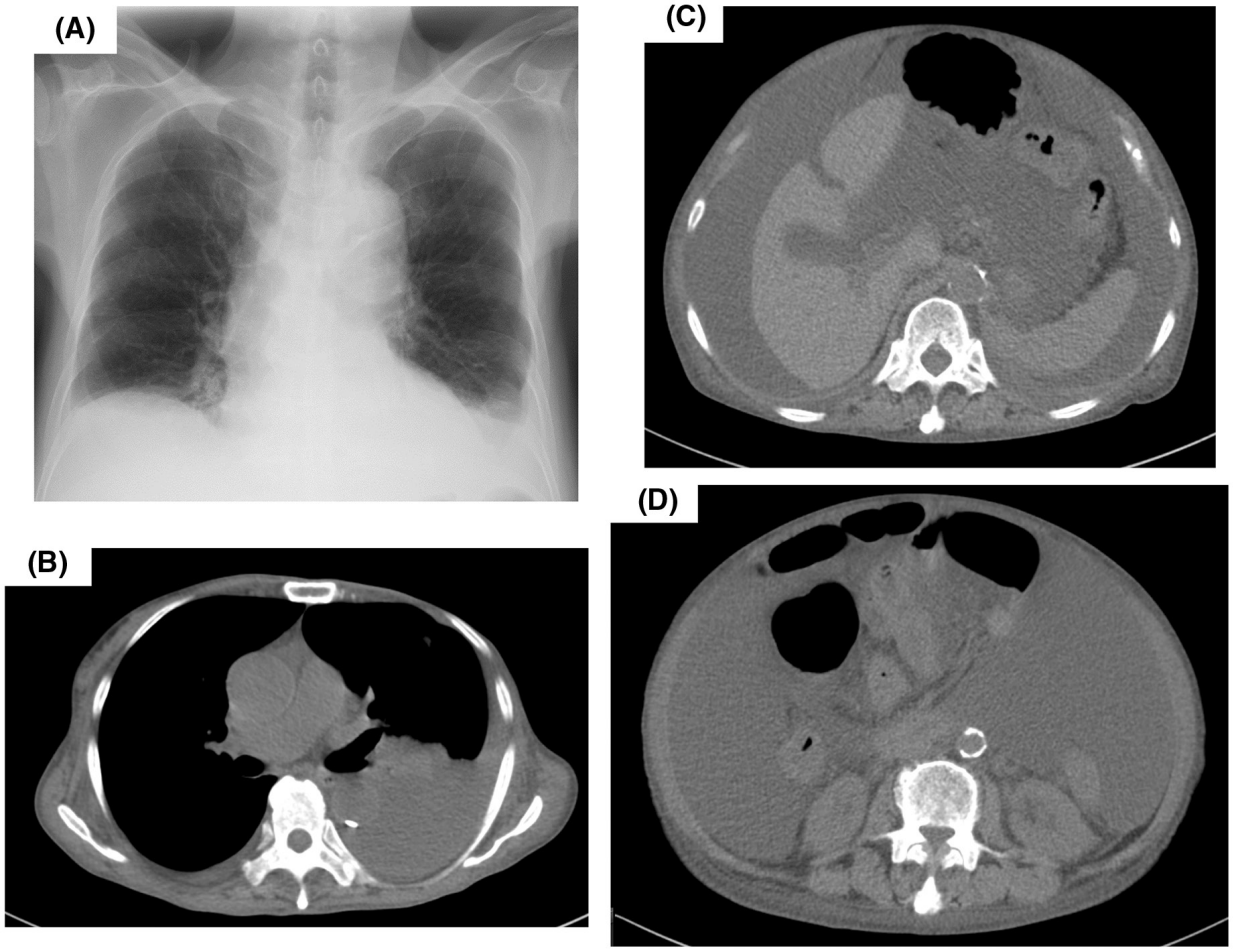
(A) Chest X‐ray (B) Chest CT on admission, exhibiting left pleural effusion. (C and D) Abdominal CT on admission, exhibiting massive ascites.
